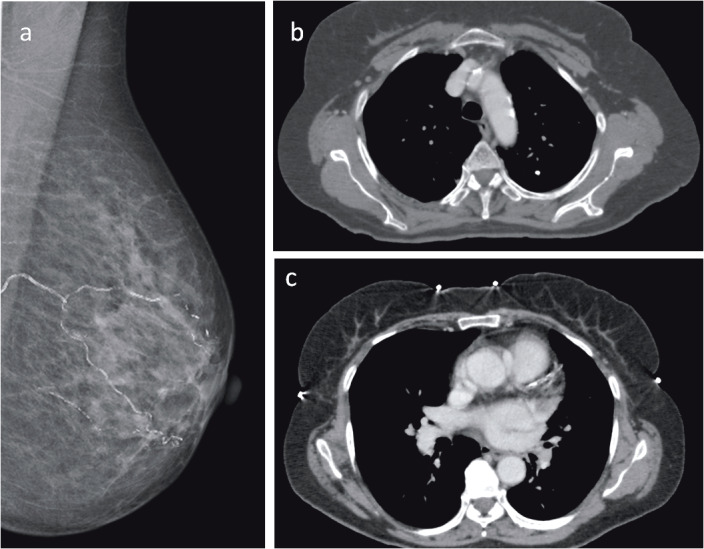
Mammography of a 62-year-old woman with grade III vascular calcification in the left breast: (a) while thoracic CT shows
